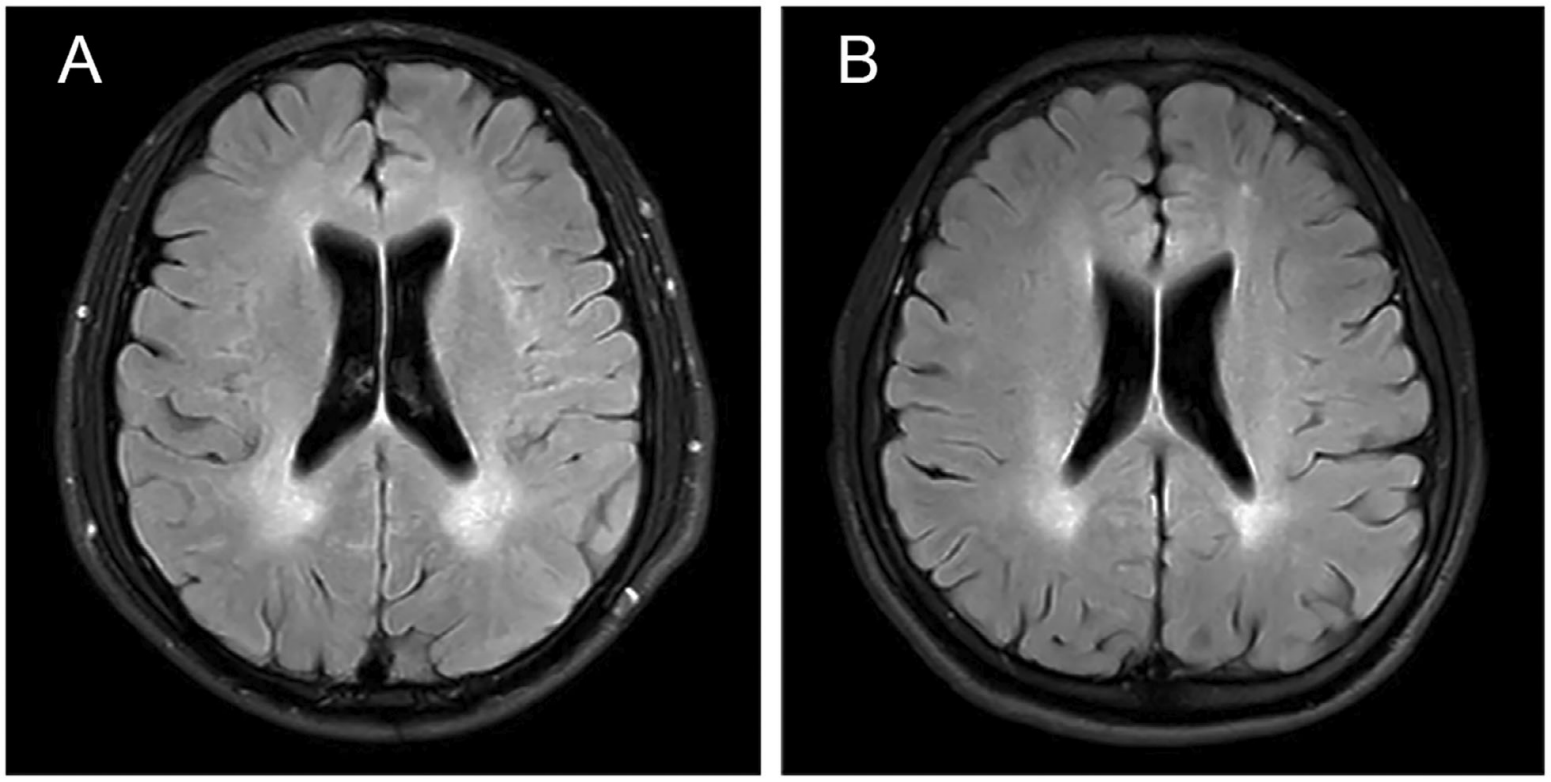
Brain MRI of patients with SPG2. (A) (patient T1866) and (B) (patient T6956) showed hypersignal intensity of white matter in periventricular regions on Flair‐weighted sequences.
